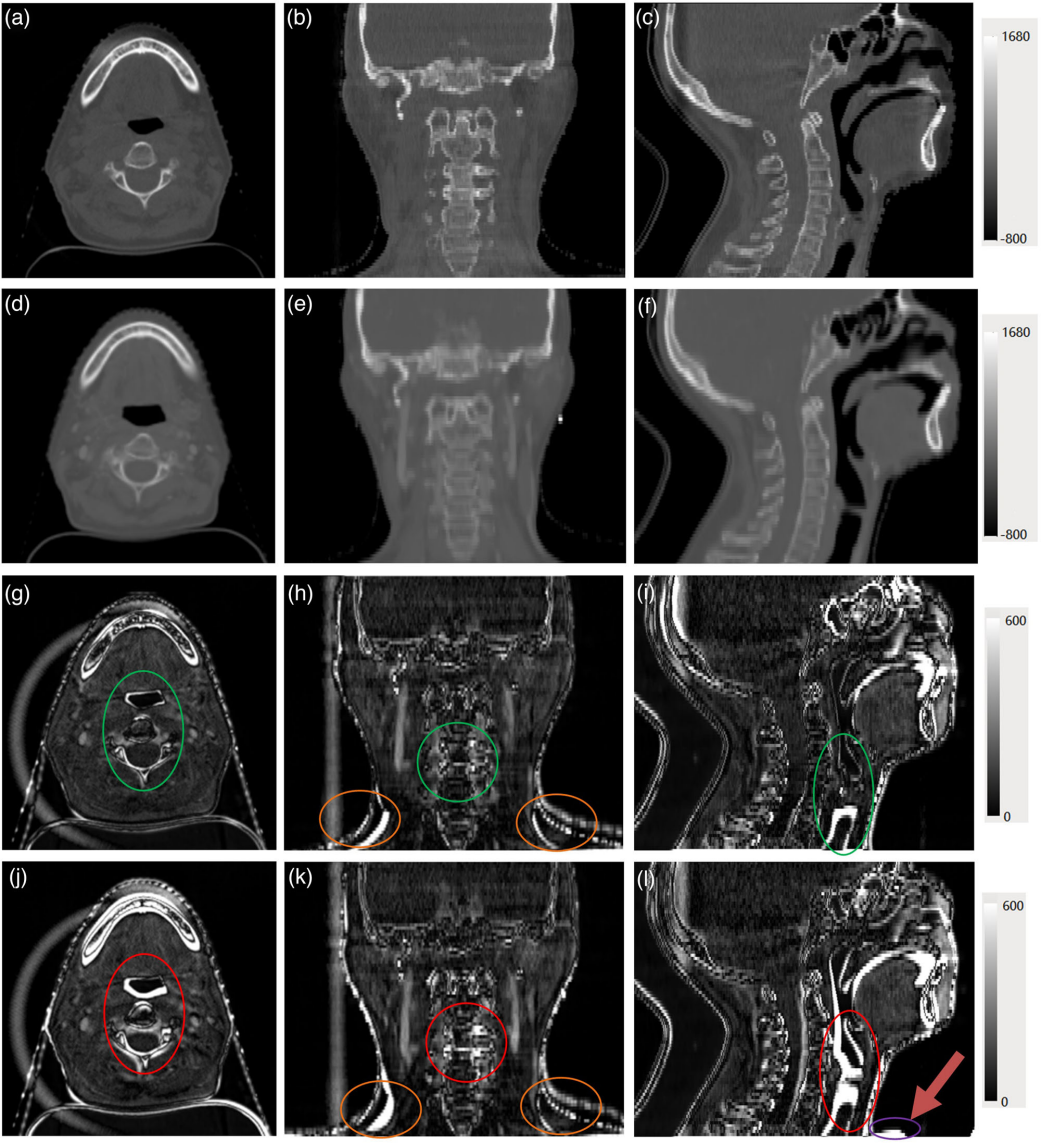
Axial, coronal, and sagittal images between registered computed tomography (CT) and cone‐beam computed tomography (CBCT) image. (a–c) CBCT, (d–f) registered CT, (g–i) difference of in‐house hybrid method, and (j–l) difference of NiftyReg
